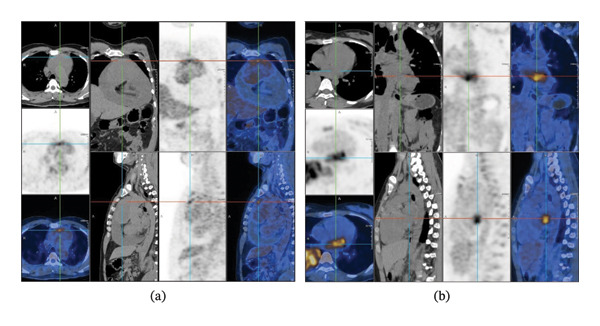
PET–CT scan of primary cardiac synovial sarcoma patients. (a) (Case 1) PET–CT scan demonstrated a heterogeneous enhancing soft tissue mass within the pericardial cavity. (b) (Case 2) PET–CT scan demonstrated a hypodense lesion with increased abnormal glucose metabolism in the left atrium and left ventricle.
